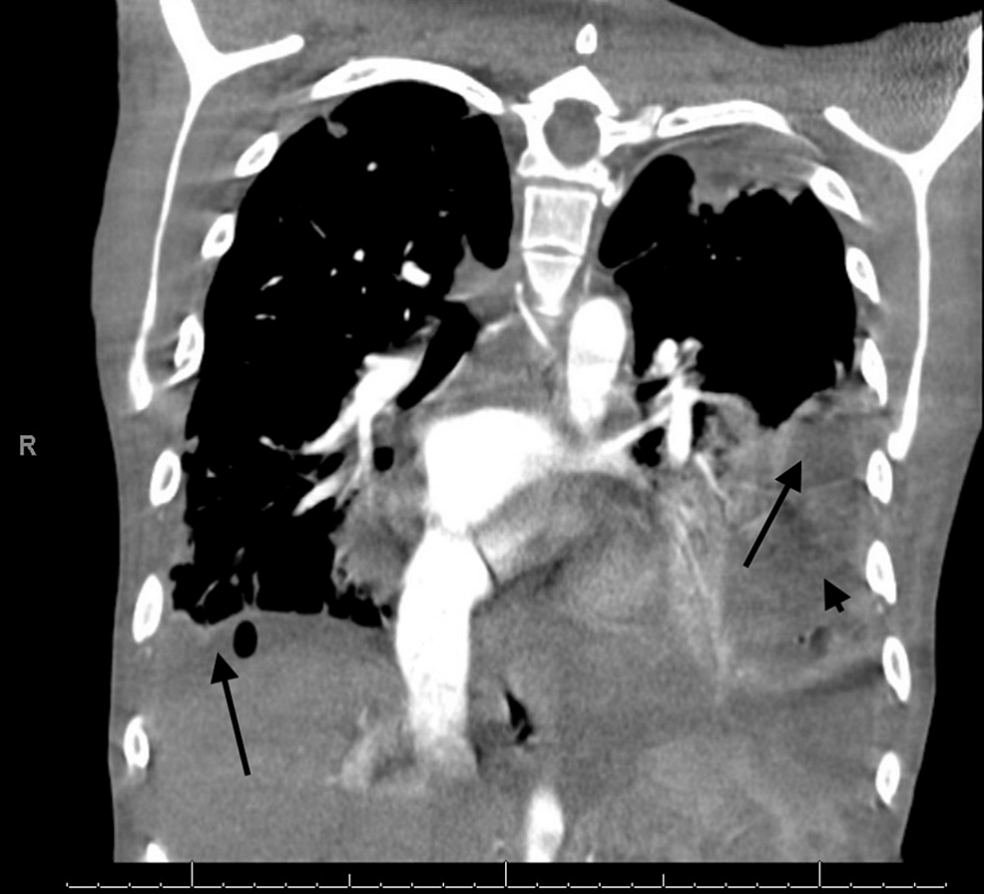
Coronal CT chest with contrast showing bilateral loculated pleural effusions (arrows) and left hydropneumothorax (arrowhead) secondary to septic pulmonary emboli.
